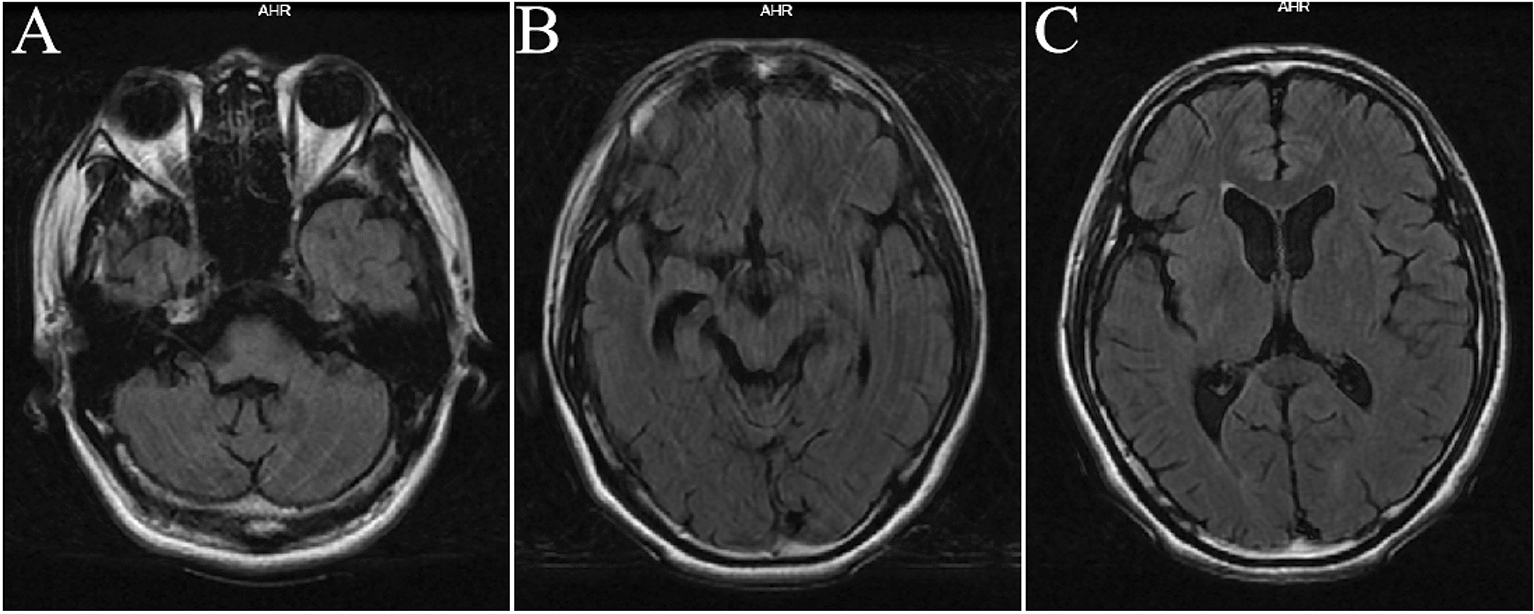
Axial T2-weighted head MRI showing hippocampus and midbrain atrophy, lateral ventricles and the third ventricle enlargement
